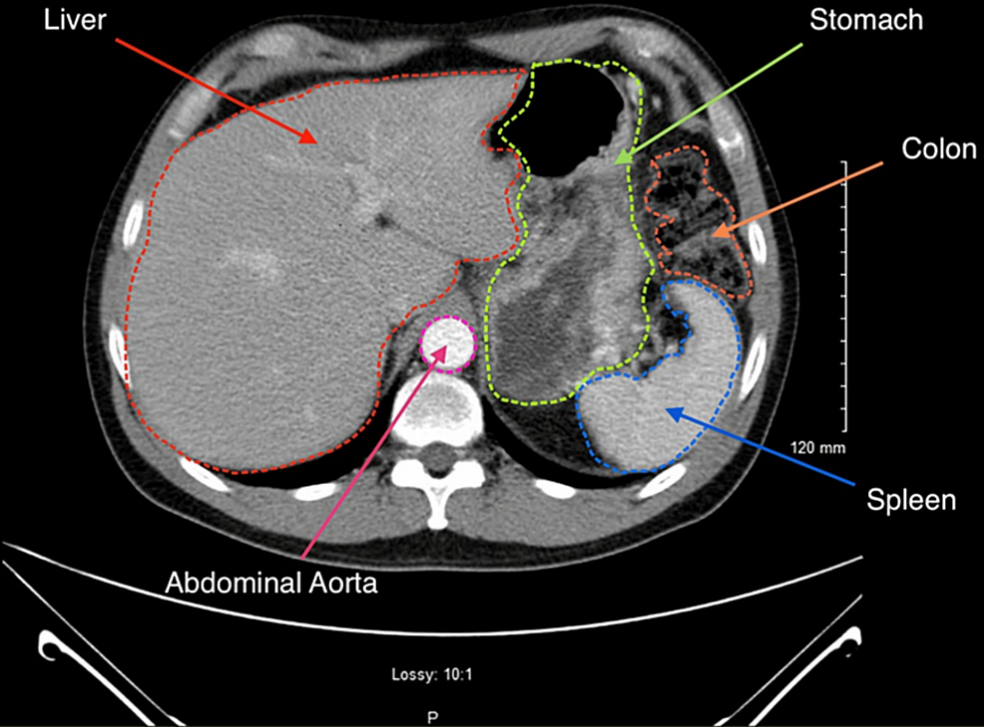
Computed tomography (CT) abdomen and pelvis with contrast The CT examination of the liver reveals an unremarkable appearance. The liver is of normal size and shape. There are no focal lesions, masses, or areas of abnormal enhancement noted within the hepatic parenchyma. The liver demonstrates homogeneous attenuation, and there are no signs of cirrhosis, fatty infiltration, or vascular abnormalities. The adjacent structures appear normal.
